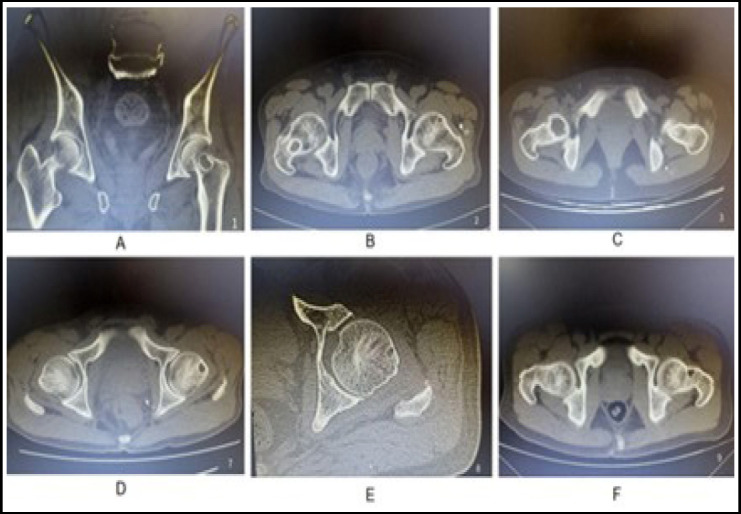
CT findings of aneurysmal bone cyst and femoral neck herniation pit. (A-C) ABCs showing expansile osteolytic destruction of the femoral neck, with cortical thinning or shell formation, heterogeneous internal density, and internal septation. (D-F) Femoral neck HPs showing small, well-circumscribed subcortical defects in the anterolateral femoral neck, with homogeneous or fluid-like internal density, thin sclerotic margins, and an occasional slit-like cortical defect.
